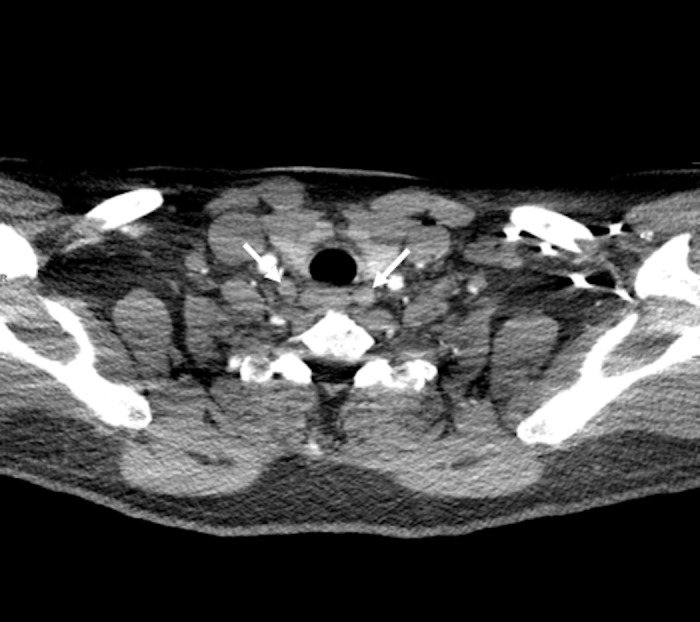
Axial Computed Tomography (CT) Scan of Parathyroid. A 57-year-old man had primary hyperparathyroidism. Preoperative parathyroid revision axial 4D-CT shows a 10 mm ovoid structure on the right and an 11 mm structure on the left side (white arrows).
